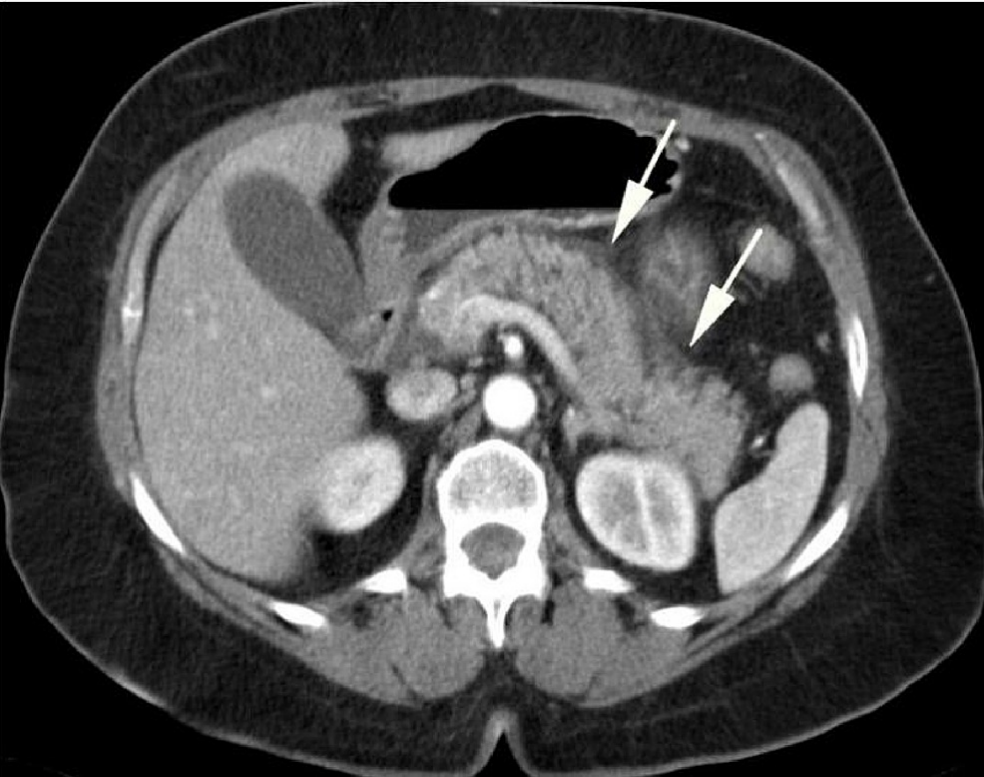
CT abdominal imaging with contrast showing acute interstitial pancreatitis (marked with an arrowhead).
